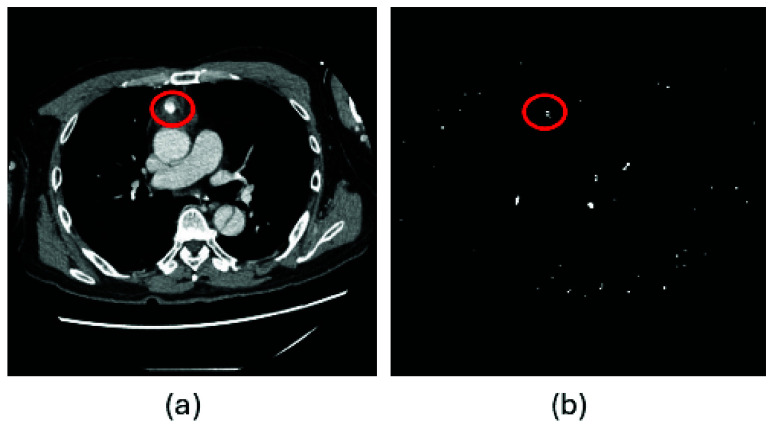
Contrast extravasation detection in a case of internal mammary artery injury. (a) Axial CT slice showing a surgeon-identified extravasation focus (circled). (b) Corresponding KGA activation map highlighting candidate regions, with the extravasation region circled and a positive alert generated.
